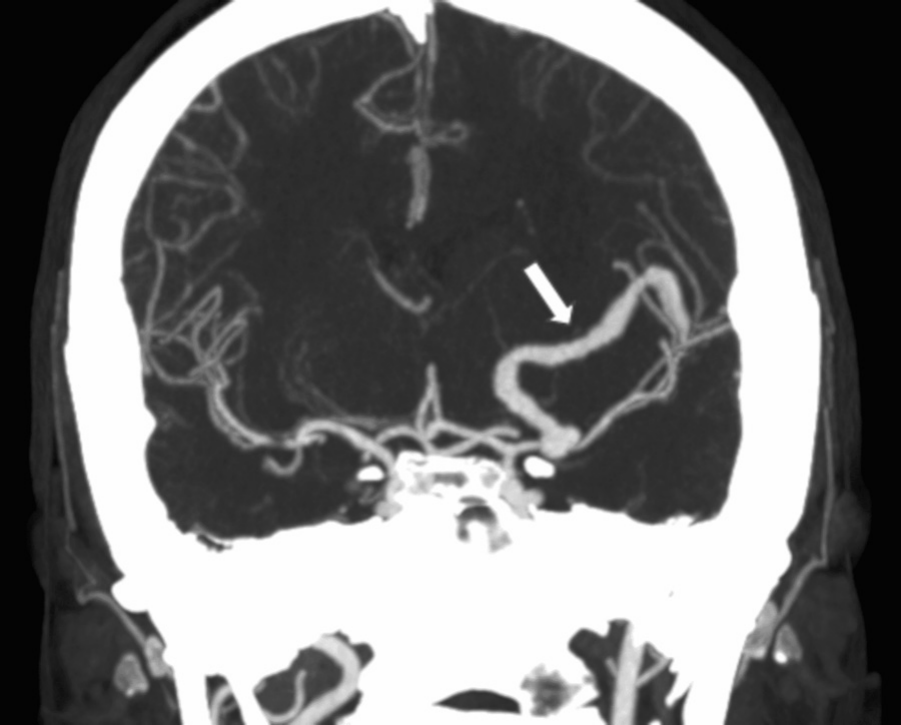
Maximum intensity projection (MIP) coronal reconstruction of a contrast-enhanced CT showing a serpentine eccentric patent vessel (arrow).
